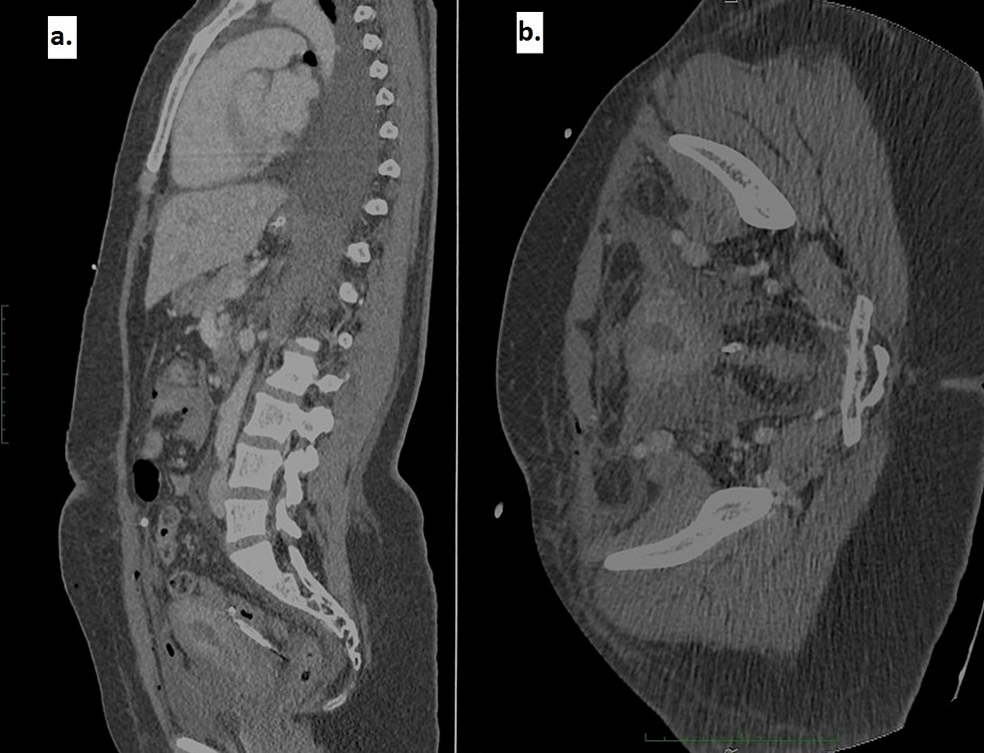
Computed tomography (CT) scan of the chest and abdomen-pelvis CT showed heterogeneous uterine density and moderate diffuse endometrial thickening.
